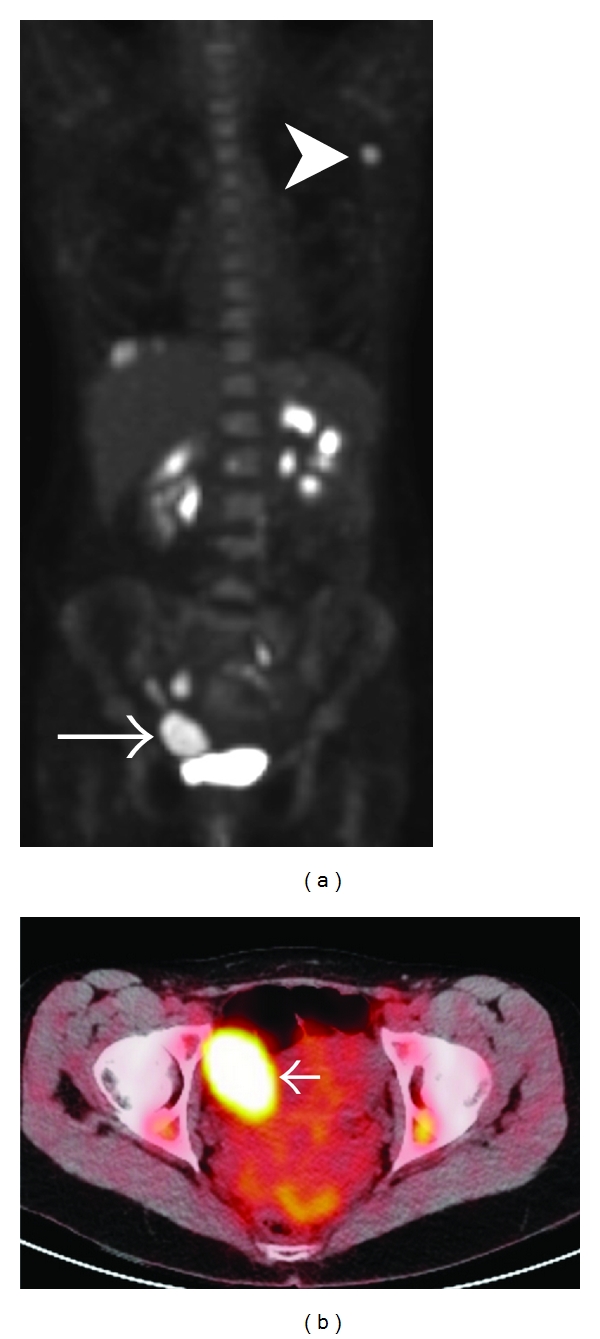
(a) Coronal maximum-intensity projection PET image shows abnormally increased FDG uptake within the left axillary lymph node (arrowhead) and within the right side of the pelvis (arrow). (b) Axial fused PET-CT image shows FDG-avid mass within the right adnexa of the pelvis, separate from the bladder (arrow).
